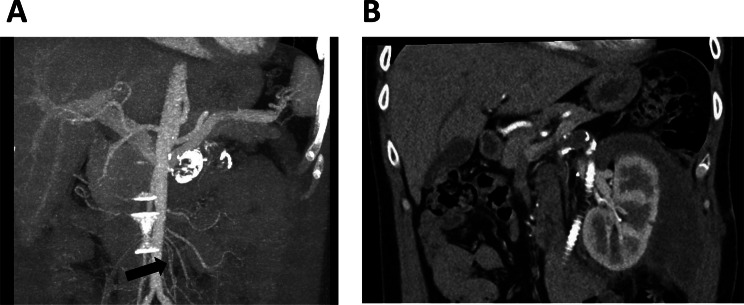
CT angiography of the abdominal aorta and its branches. A – Occlusion of the left aortal-renal bypass with calcification of the vein graft. Additionally, well-developed collateral circulation is indicated (black arrow). B – Recovery of perfusion in the left kidney following the placement of aorto-renal ePTFE (expanded polytetrafluoroethylene) vascular graft
